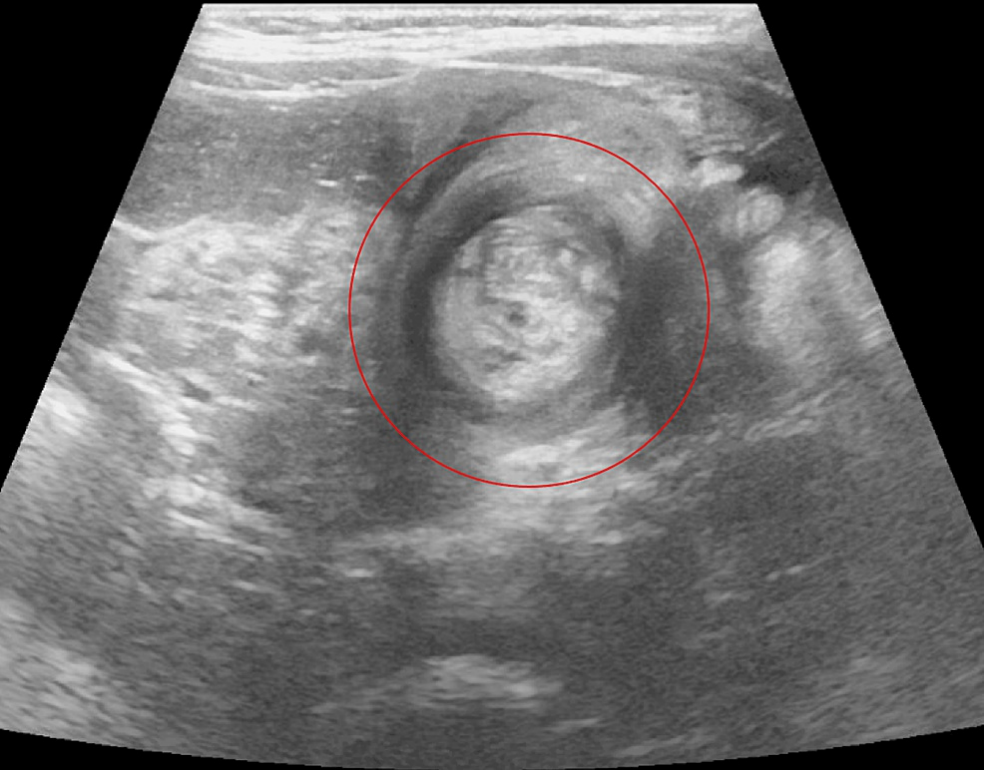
Ultrasound findings of intussusception Intussusception measures at least 3 cm in cross-section, suggesting a colonic component, most likely ileocolic. Findings compatible with intussusception were favored to be ileocolic. The telescoping bowel is circled in red.
